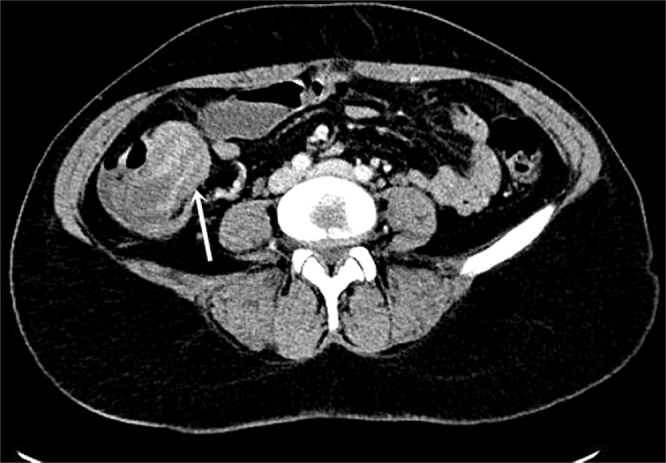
Axial contrast-enhanced CT image demonstrating the classic “target sign” (white arrow) of an ileocecal intussusception, which prompted urgent surgical exploration.
